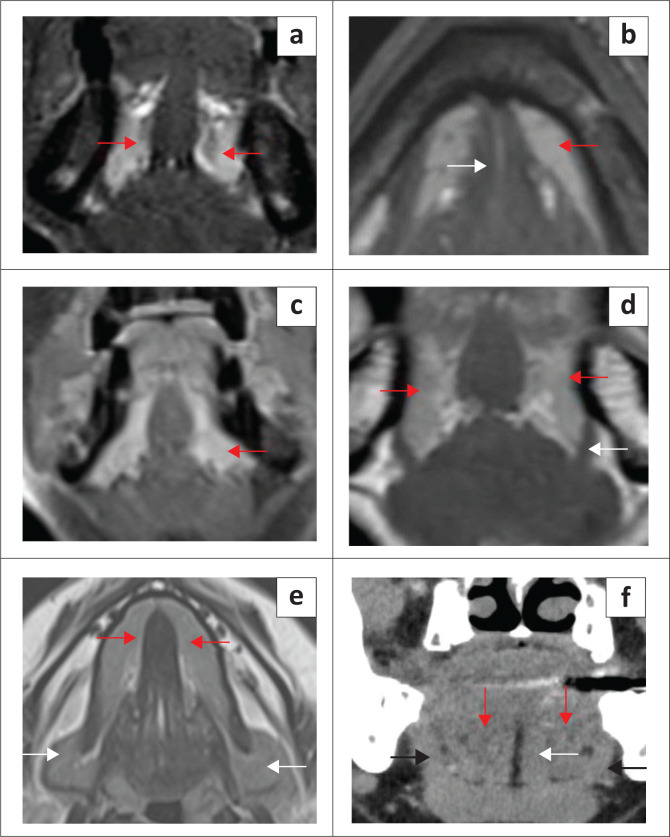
Anatomy of the sublingual space. T2 fat-suppressed coronal (a), T2 axial (b), T1 contrast-enhanced fat-suppressed (c) and T1 coronal without fat-suppression (d) show the sublingual glands (red arrows), mylohyoid muscle (d, white arrow), geniohyoid (b, white arrow). T2 axial (e) demonstrates the deep part of submandibular glands (white arrows) in floor of the mouth (FOM), posterior to the mylohyoid muscles and sublingual glands (red arrows). CT coronal (f) shows the mixed fat density of sublingual glands (red arrows) between the genioglossus (white arrow) and mylohyoid muscles (black arrows).
